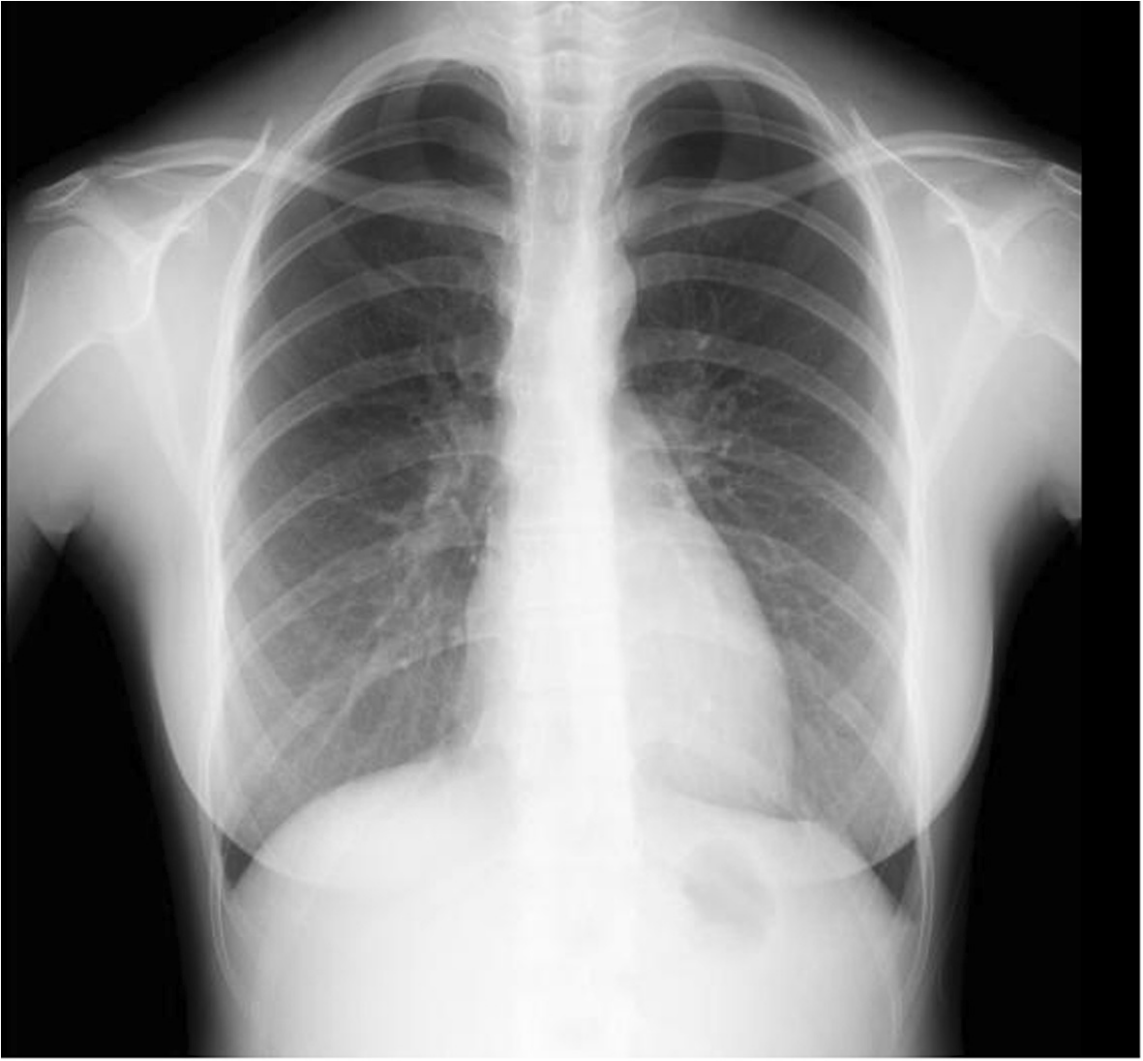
Chest X-ray showed bilateral pneumothorax in a health screening.
